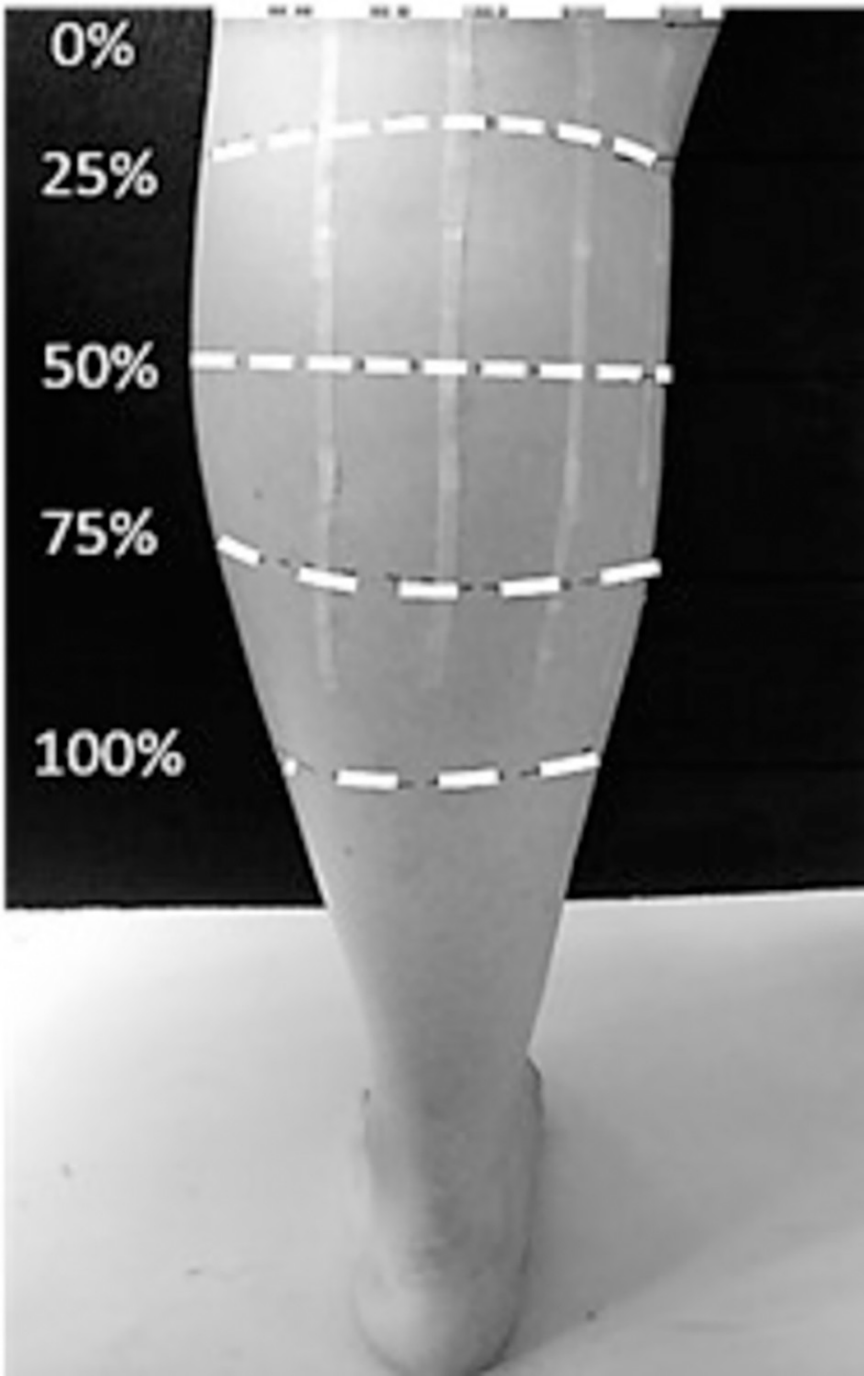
Marking on the lower limb in view of performing ultrasound scanning.
